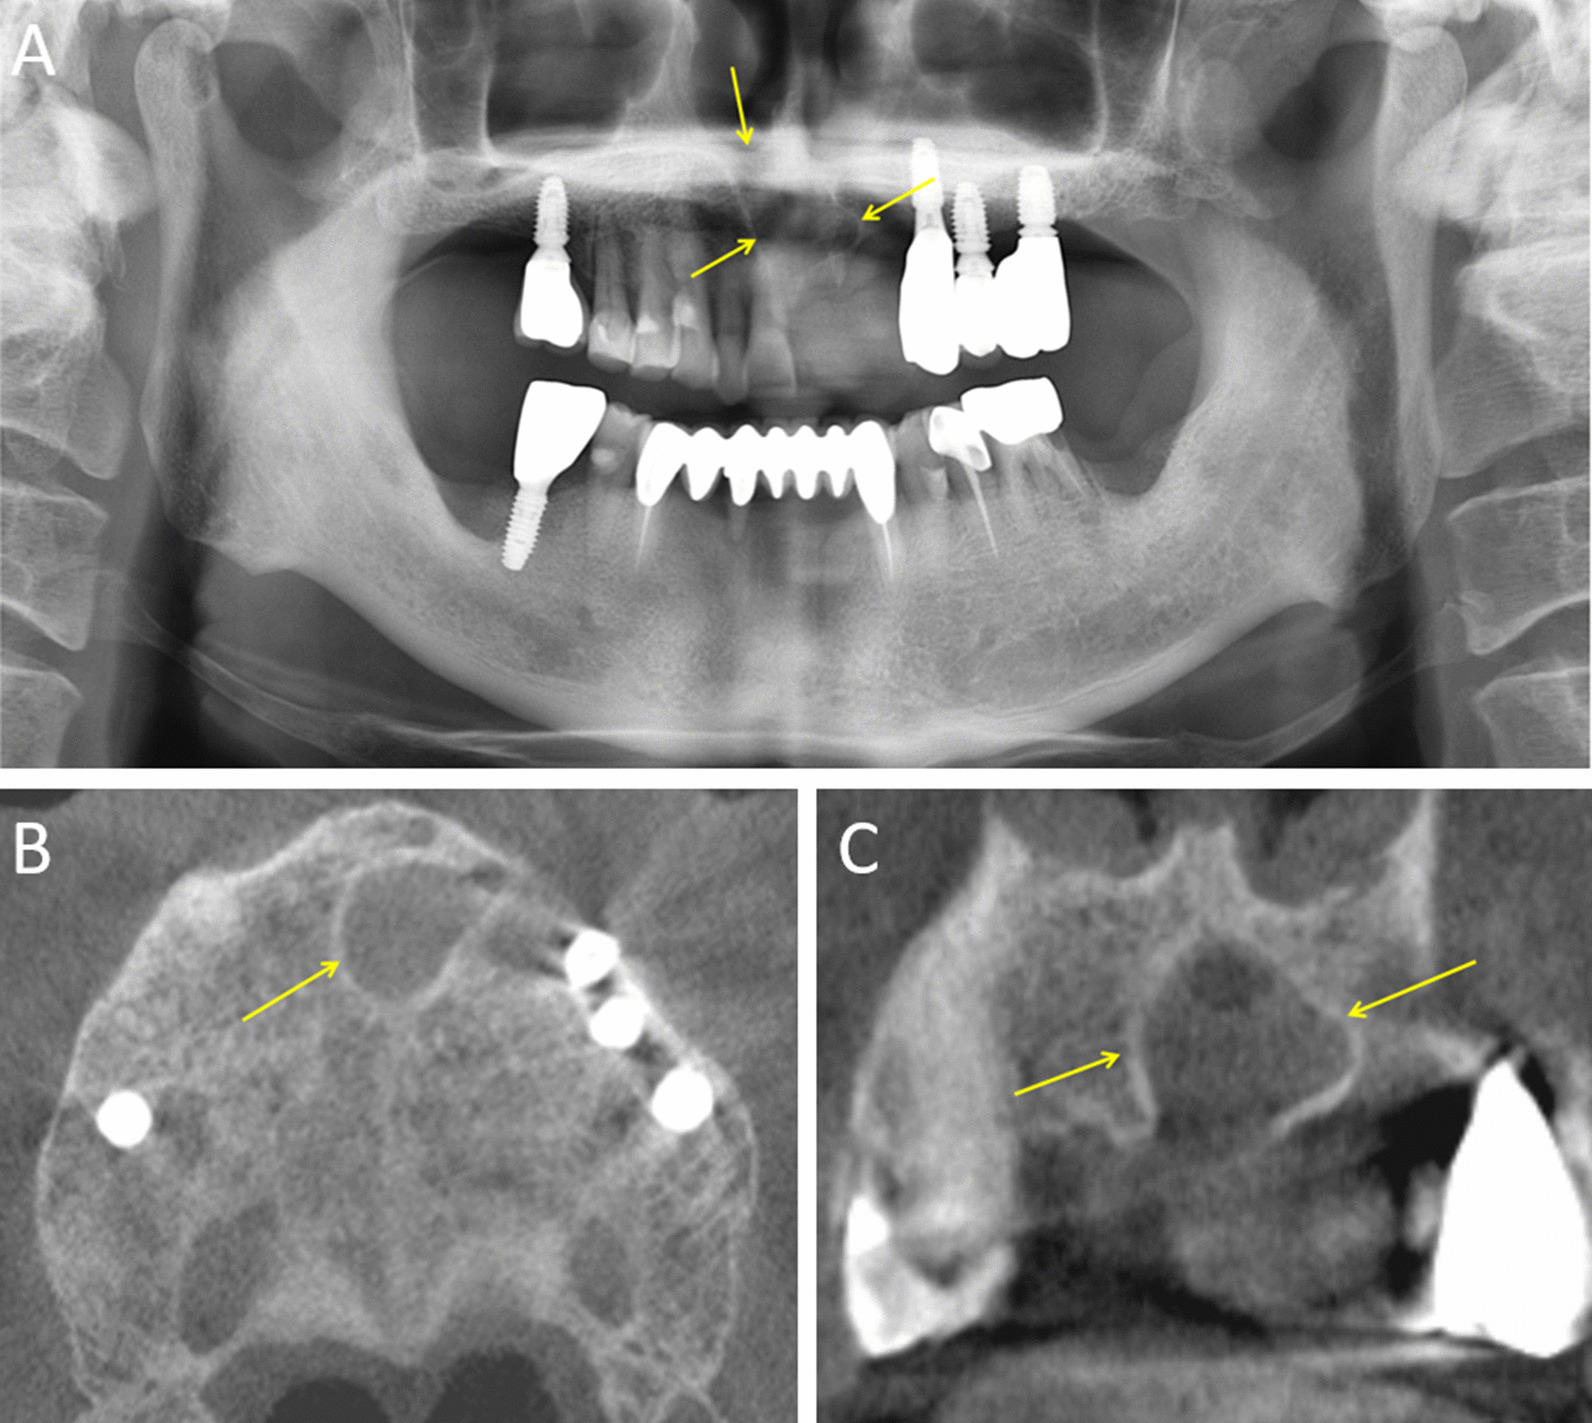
a Panoramic radiograph showing a radiolucent lesion in the anterior maxilla (yellow arrow). b, c Cone-beam computed tomography scan showing a well-defined corticated cystic lesion. (B. axial, C. coronal)
